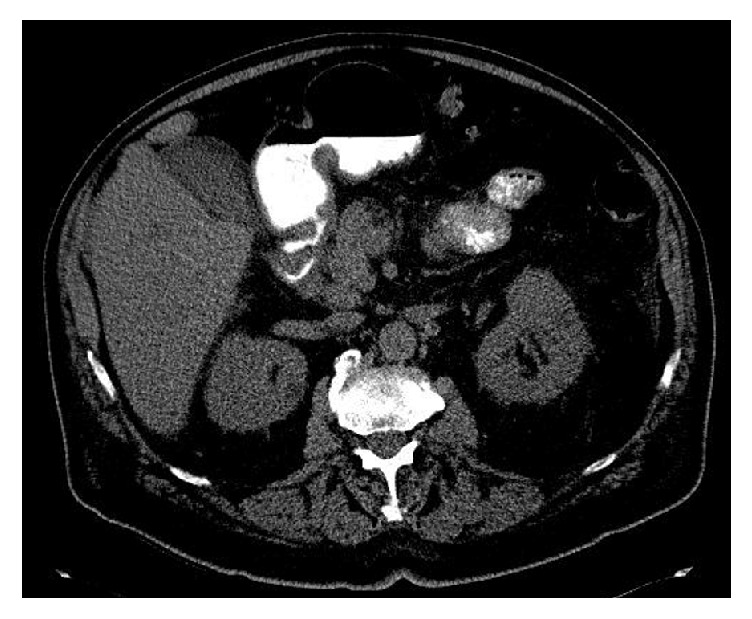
Axial unenhanced CT of the abdomen obtained at presentation shows a diffusely thickened and low attenuation gallbladder wall.
